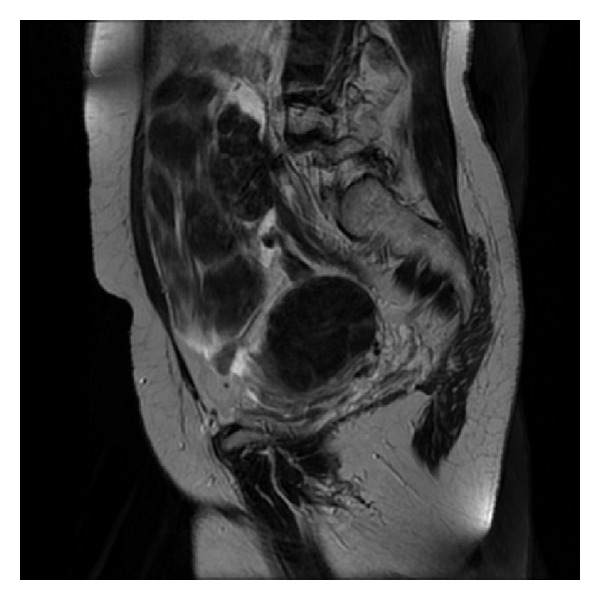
Magnetic resonance imaging (MRI) of the abdomen revealing a solid mass 20 cm of maximum diameter.
